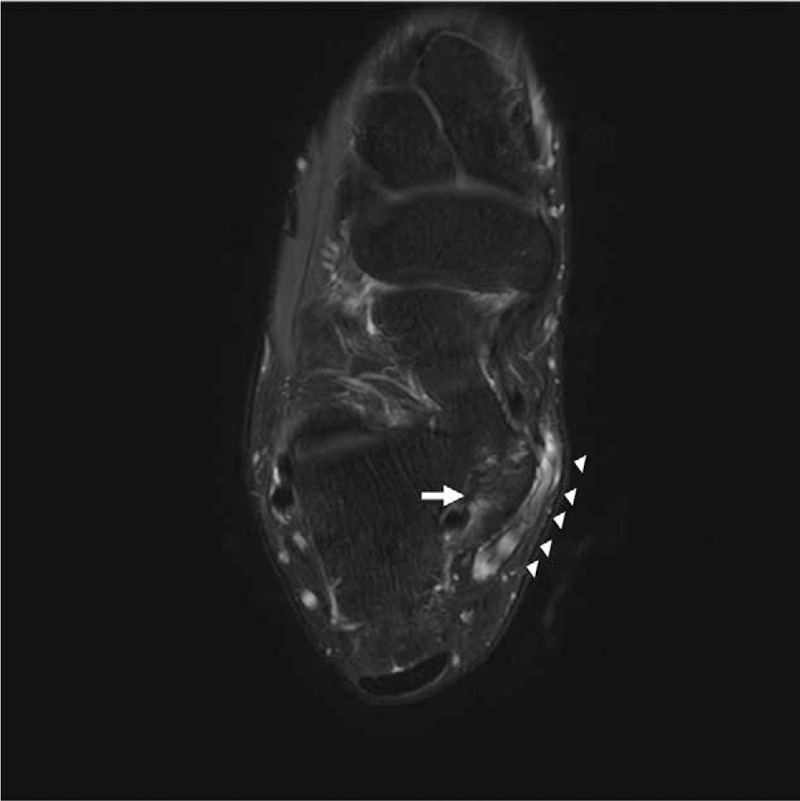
Preoperative axial, T2-weighted magnetic resonance image of the right ankle showing the accessory ossicle (arrow) in a close relationship with the sustentaculum tali. Mild bone marrow edema is noted in the ossicle, and the displaced medial tarsal structures are revealed (arrowheads).
